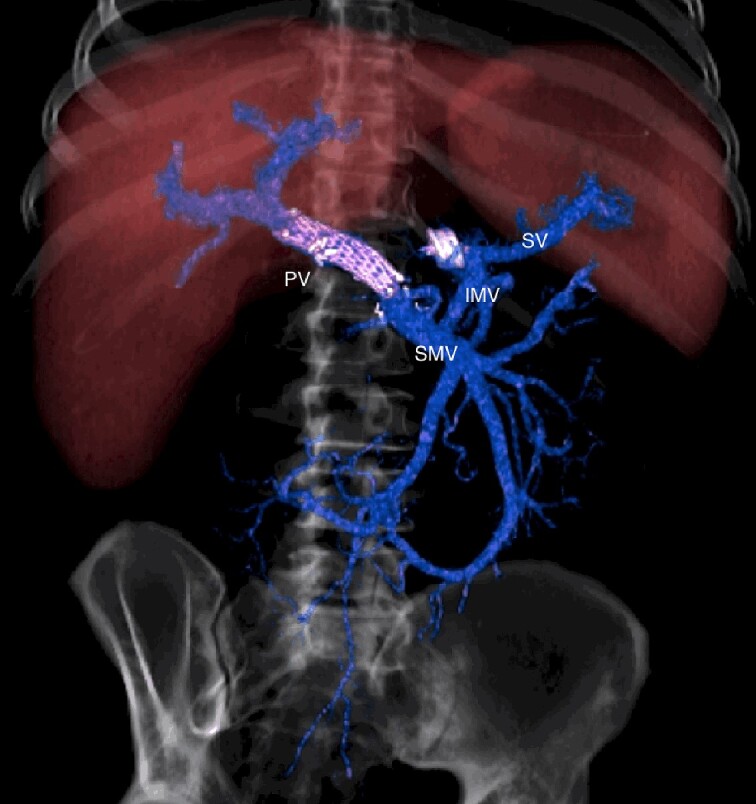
CT scan after other interventional radiology with plug in the splenic vein and cover stent in the superior mesenteric vein. PV portal vein, SMV superior mesenteric vein, SV splenic vein, IMV inferior mesenteric vein.
